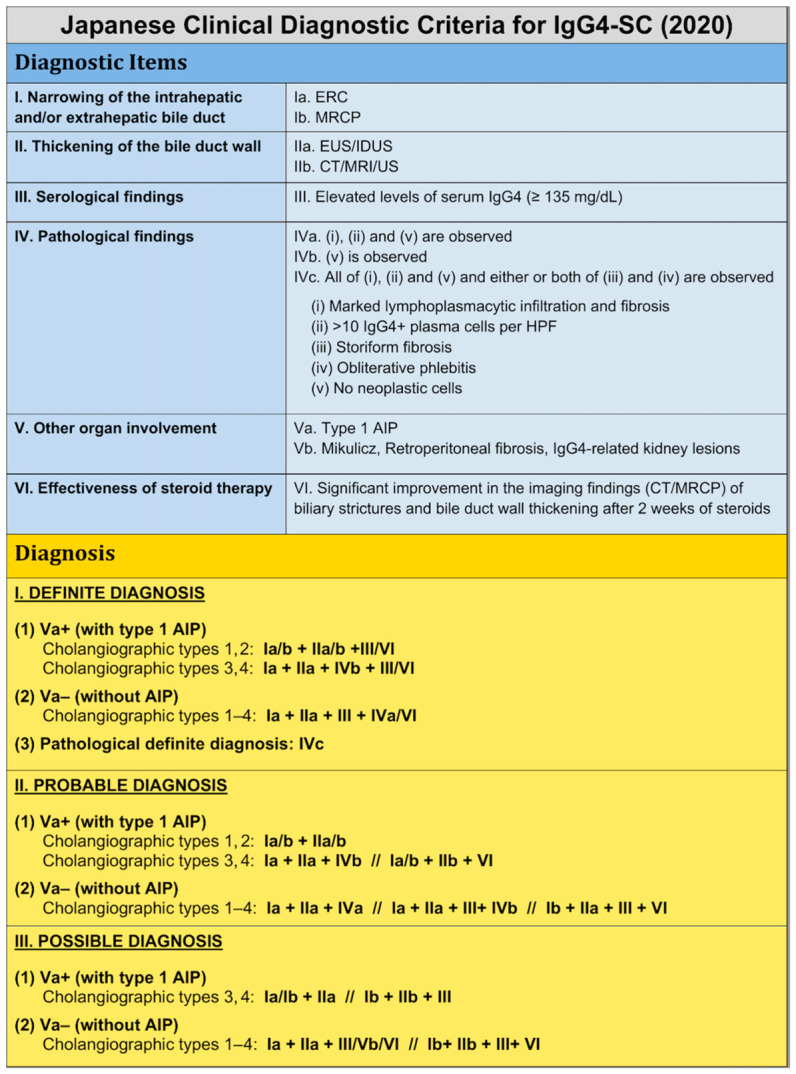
Japanese clinical diagnostic criteria for IgG4-SC (2020). Adapted from Nakazawa et al. [57] and Naitoh et al. [58]. According to these criteria, patients are first classified as “with AIP” or “without AIP”, after which cholangiographic patterns are used to differentiate IgG4-SC from primary sclerosing cholangitis and cholangiocarcinoma. ERC = endoscopic retrograde cholangiography; MRCP = magnetic resonance cholangiopancreatography; EUS = endoscopic ultrasound; IDUS = intraductal ultrasound; AIP = autoimmune pancreatitis; HPF = high-power field.
